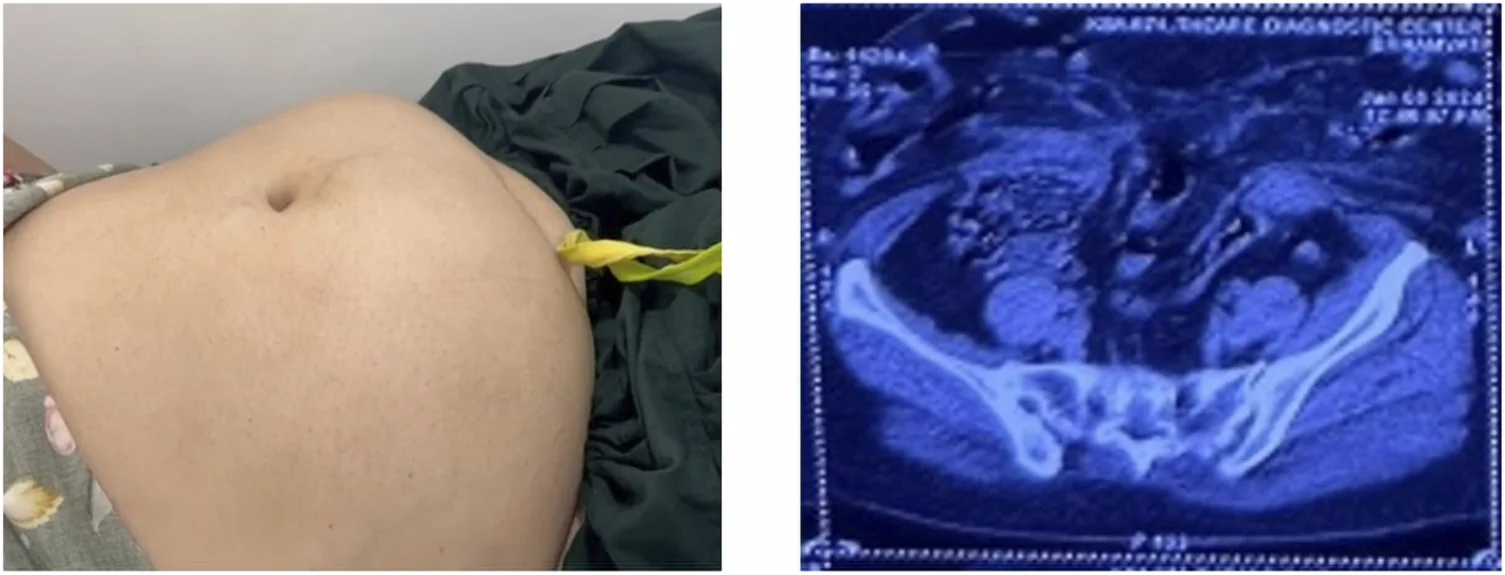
Shows clinical and radiological presentation of a complex ventral hernia. Axial CT scan (right) shows a 10.5 × 13.5 cm abdominal wall defect with loss of domain containing mesenteric fat and bowel loops, corresponding to the clinical mass seen in the patient photograph (left).
